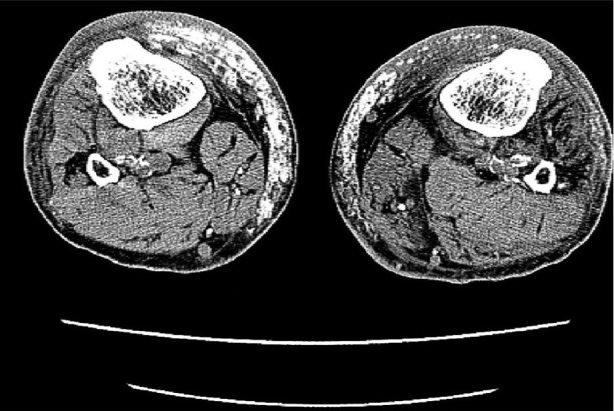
CT images of lower extremities showed calcified vessels and subcutaneous calcification
